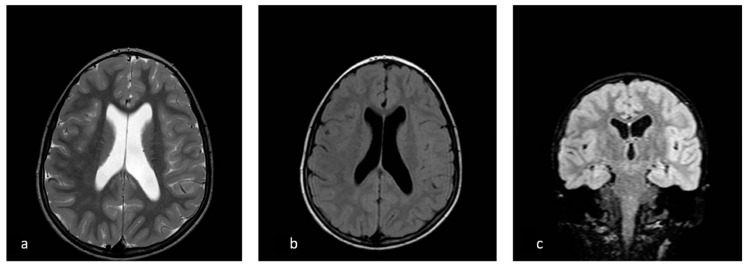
Ventricular asymmetry (left > right) in axial T2 weighted sequence (a), axial T2 FLAIR sequence (b), and coronal multi planar rendering (c).
